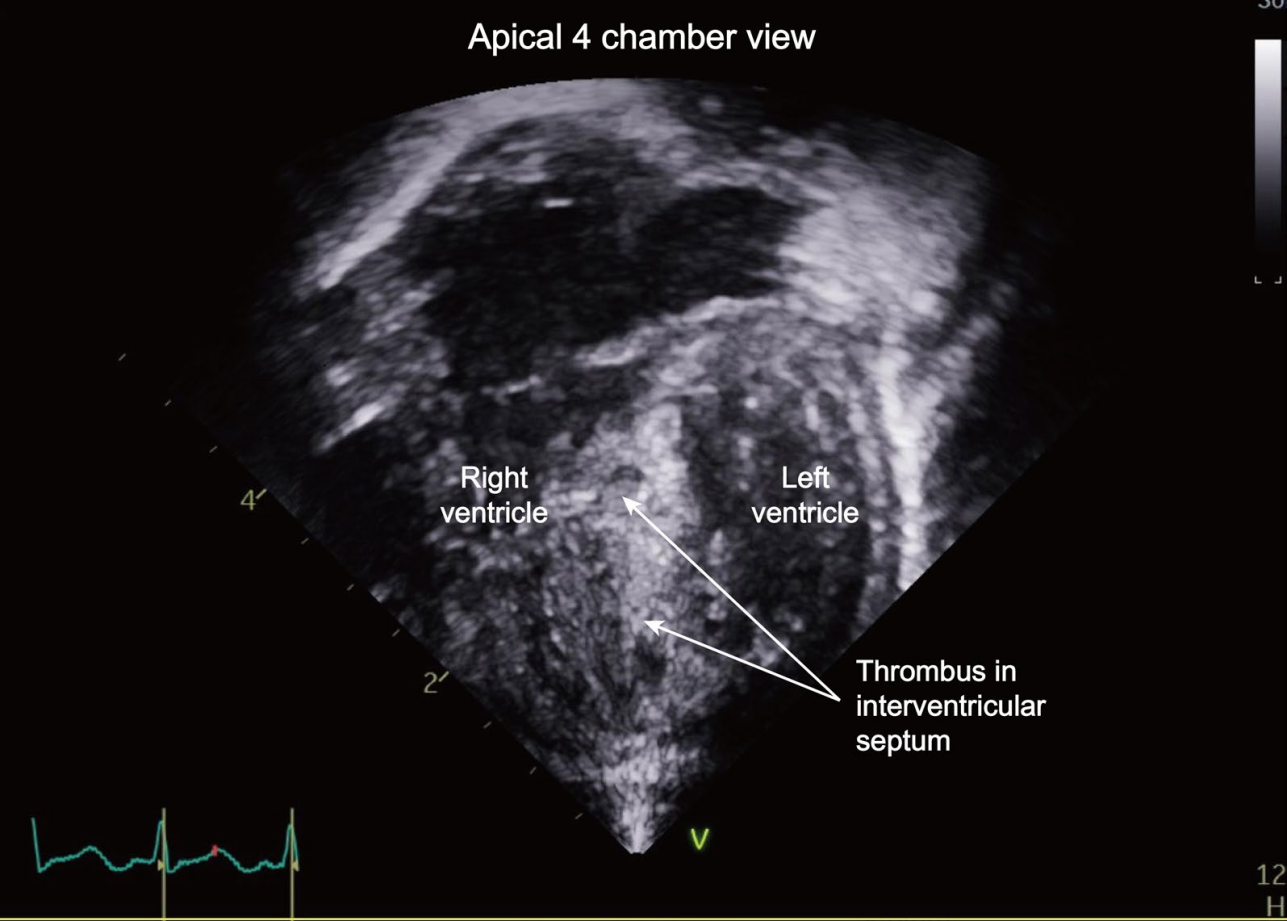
Follow-up transthoracic echocardiography showing a decrease in the size of the anechoic interventricular septal hematomas with thrombi filling the hematomas in the apical 4-chamber view on postoperative day 4. (Additional file 3: Video S3)
